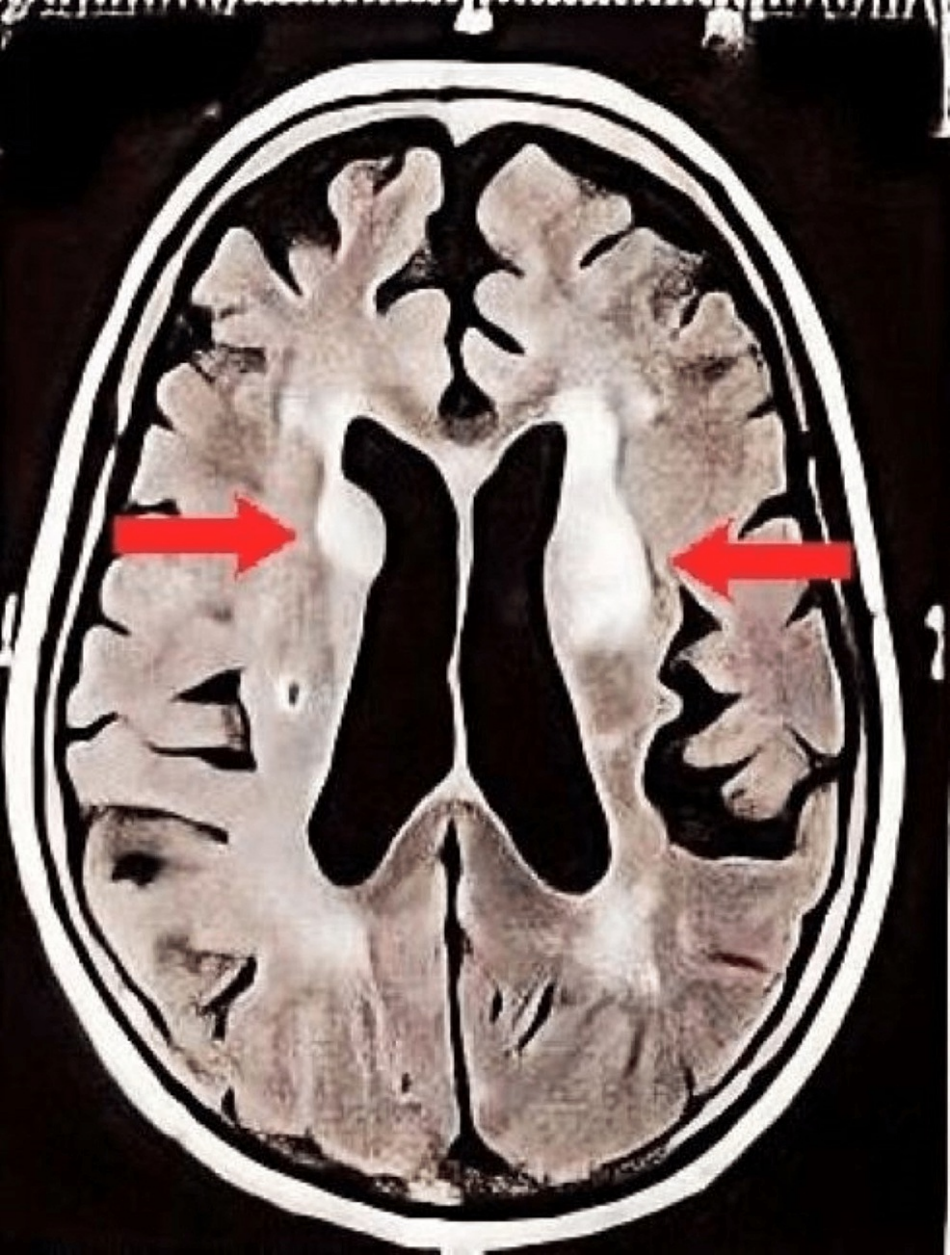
T2W MRI with contrast in Case 3 showing atrophy and microvascular ischemic changes; hyperintensity in bilateral basal ganglia regions suggestive of infarct (red arrow). T2W: T2 weighted
